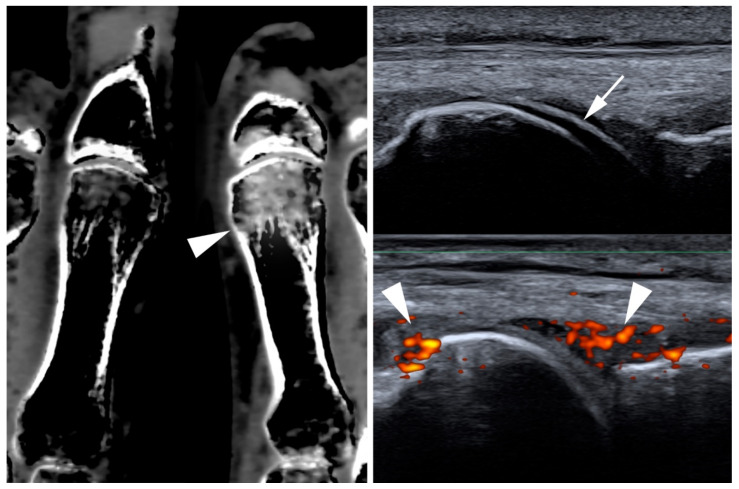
Multimodality imaging for gout. (Left): Virtual calcium subtraction imaging from dual-energy computed tomography. The arrowhead indicates bone marrow edema in the first metacarpal head. (Right): Ultrasound image of the same patient. The arrow indicates double-contour sign and arrowheads indicate synovitis on the power Doppler.
